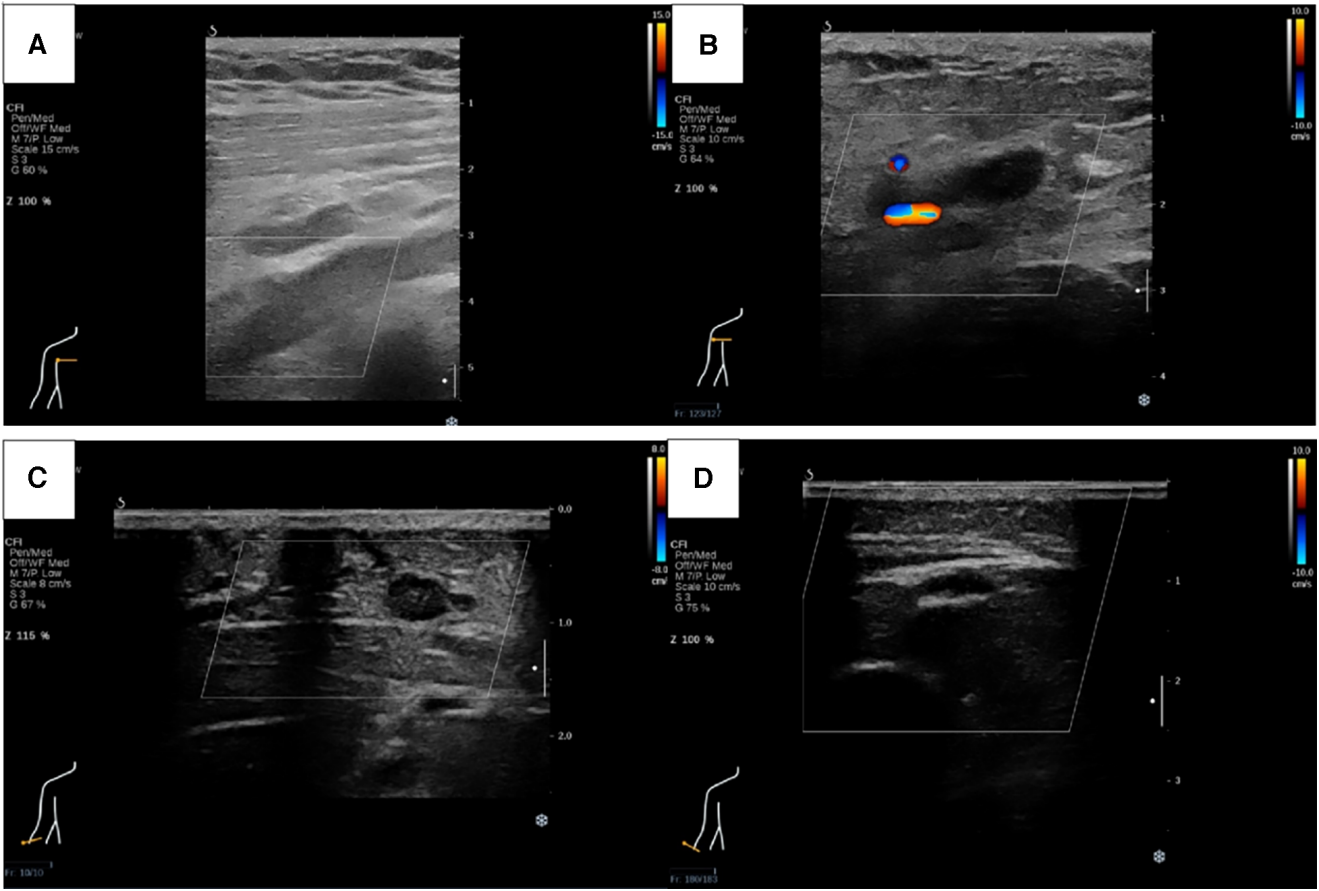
Ultrasound revealed thrombosis of right subclavian vein (A), axillary vein (B) and cephalic vein (C), as well as right radial, ulnar and part of branchial artery (D).
